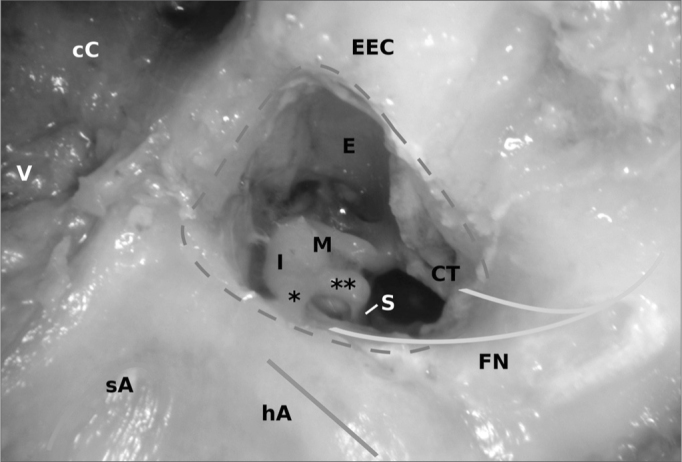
A mastoidectal approach to a lambs' middle ear (right ear). The bony labyrinth block is enclosed by cranial cells (cC) containing fat and a spongiform structured skull occipital and caudal. The axis of the lateral (blue) and the superior (green)  semicircular canal are made visible. The access to middle ear is artificial by a partial resection of the external ear canal. The stitched grey line shows the margin of this opening. The facial nerve (FN) encircles the stapes (S) as in humans and serves the chorda tympani (CT). The incus (I) is to be found medial of the lateral arcade. M = Malleus, E = Epitympanon, V = venous vessel, * = short process,  = long process.
